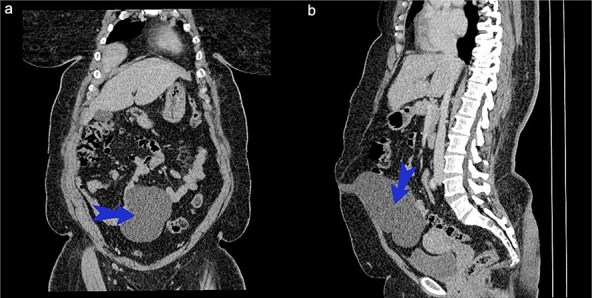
(a) CT scan of abdomen coronary view. (b) CT scan of abdomen sagittal view.
